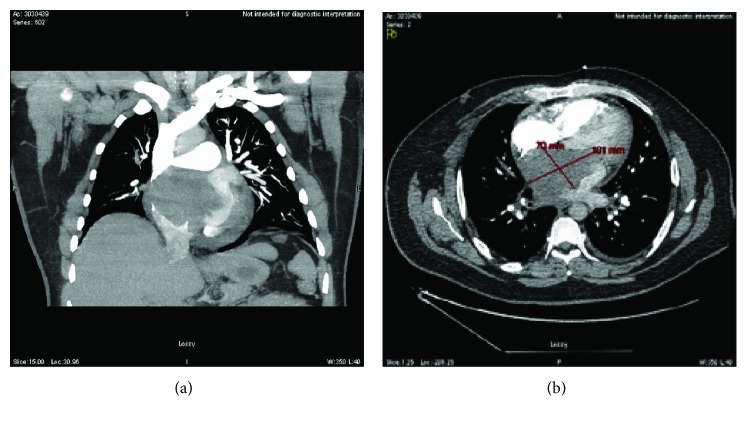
Computed tomography of the chest demonstrating the mass in the left atrium.
